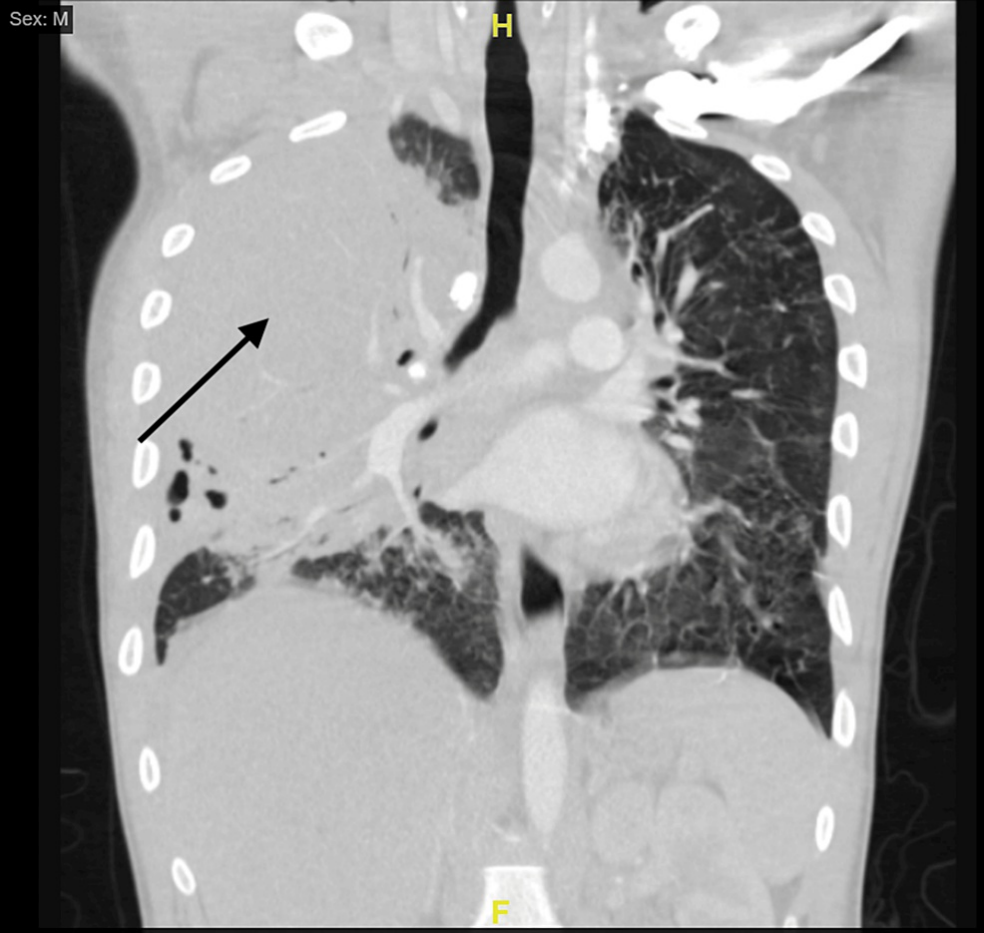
Computed tomography scan showing right-sided infiltrate with fibrosis and chronic granulomatous calcifications
